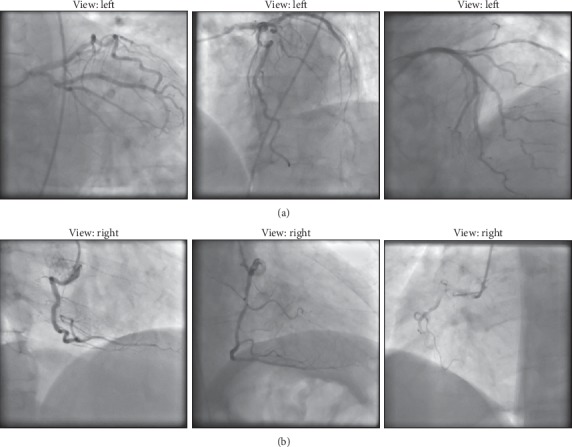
X-ray coronary angiography training samples.
